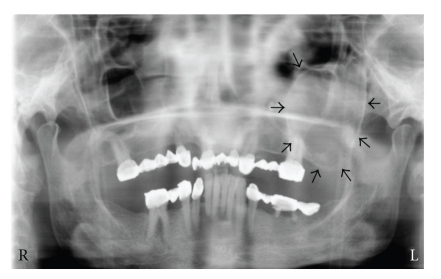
A unilocular well-defined radiolucency on orthopantomography.
